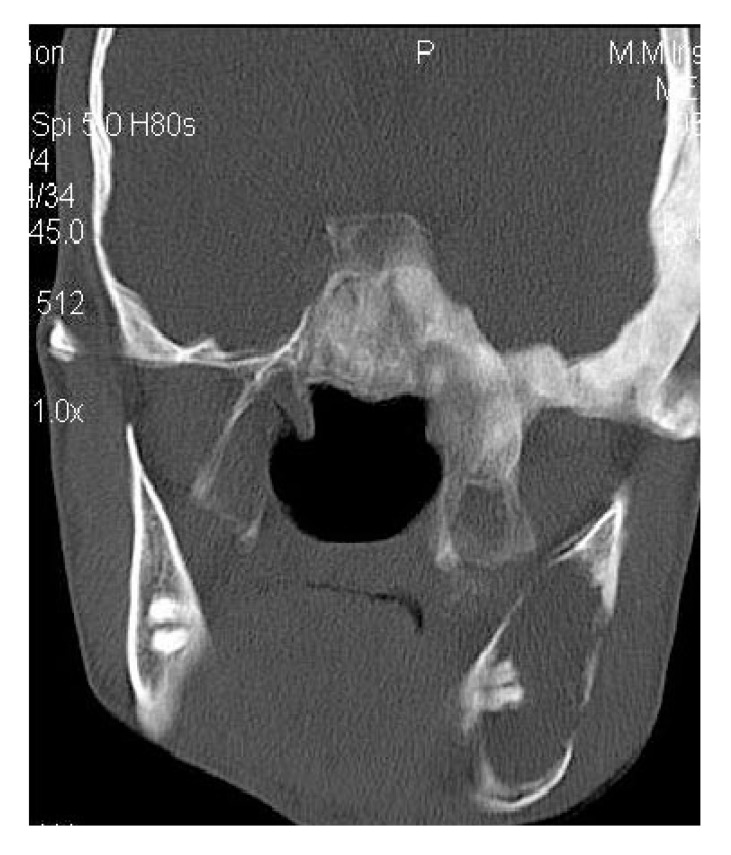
Coronal CT section showing the involvement of the zygomatic arch, zygoma, base of the cranium, mastoid process, pterygoid plates and sphenoid of right side. There was evidence of involvement of occipital condyles of both the sides and clivus with evidence of a cystic lesion in the right mandible extending from body of the mandible to the ramus.
